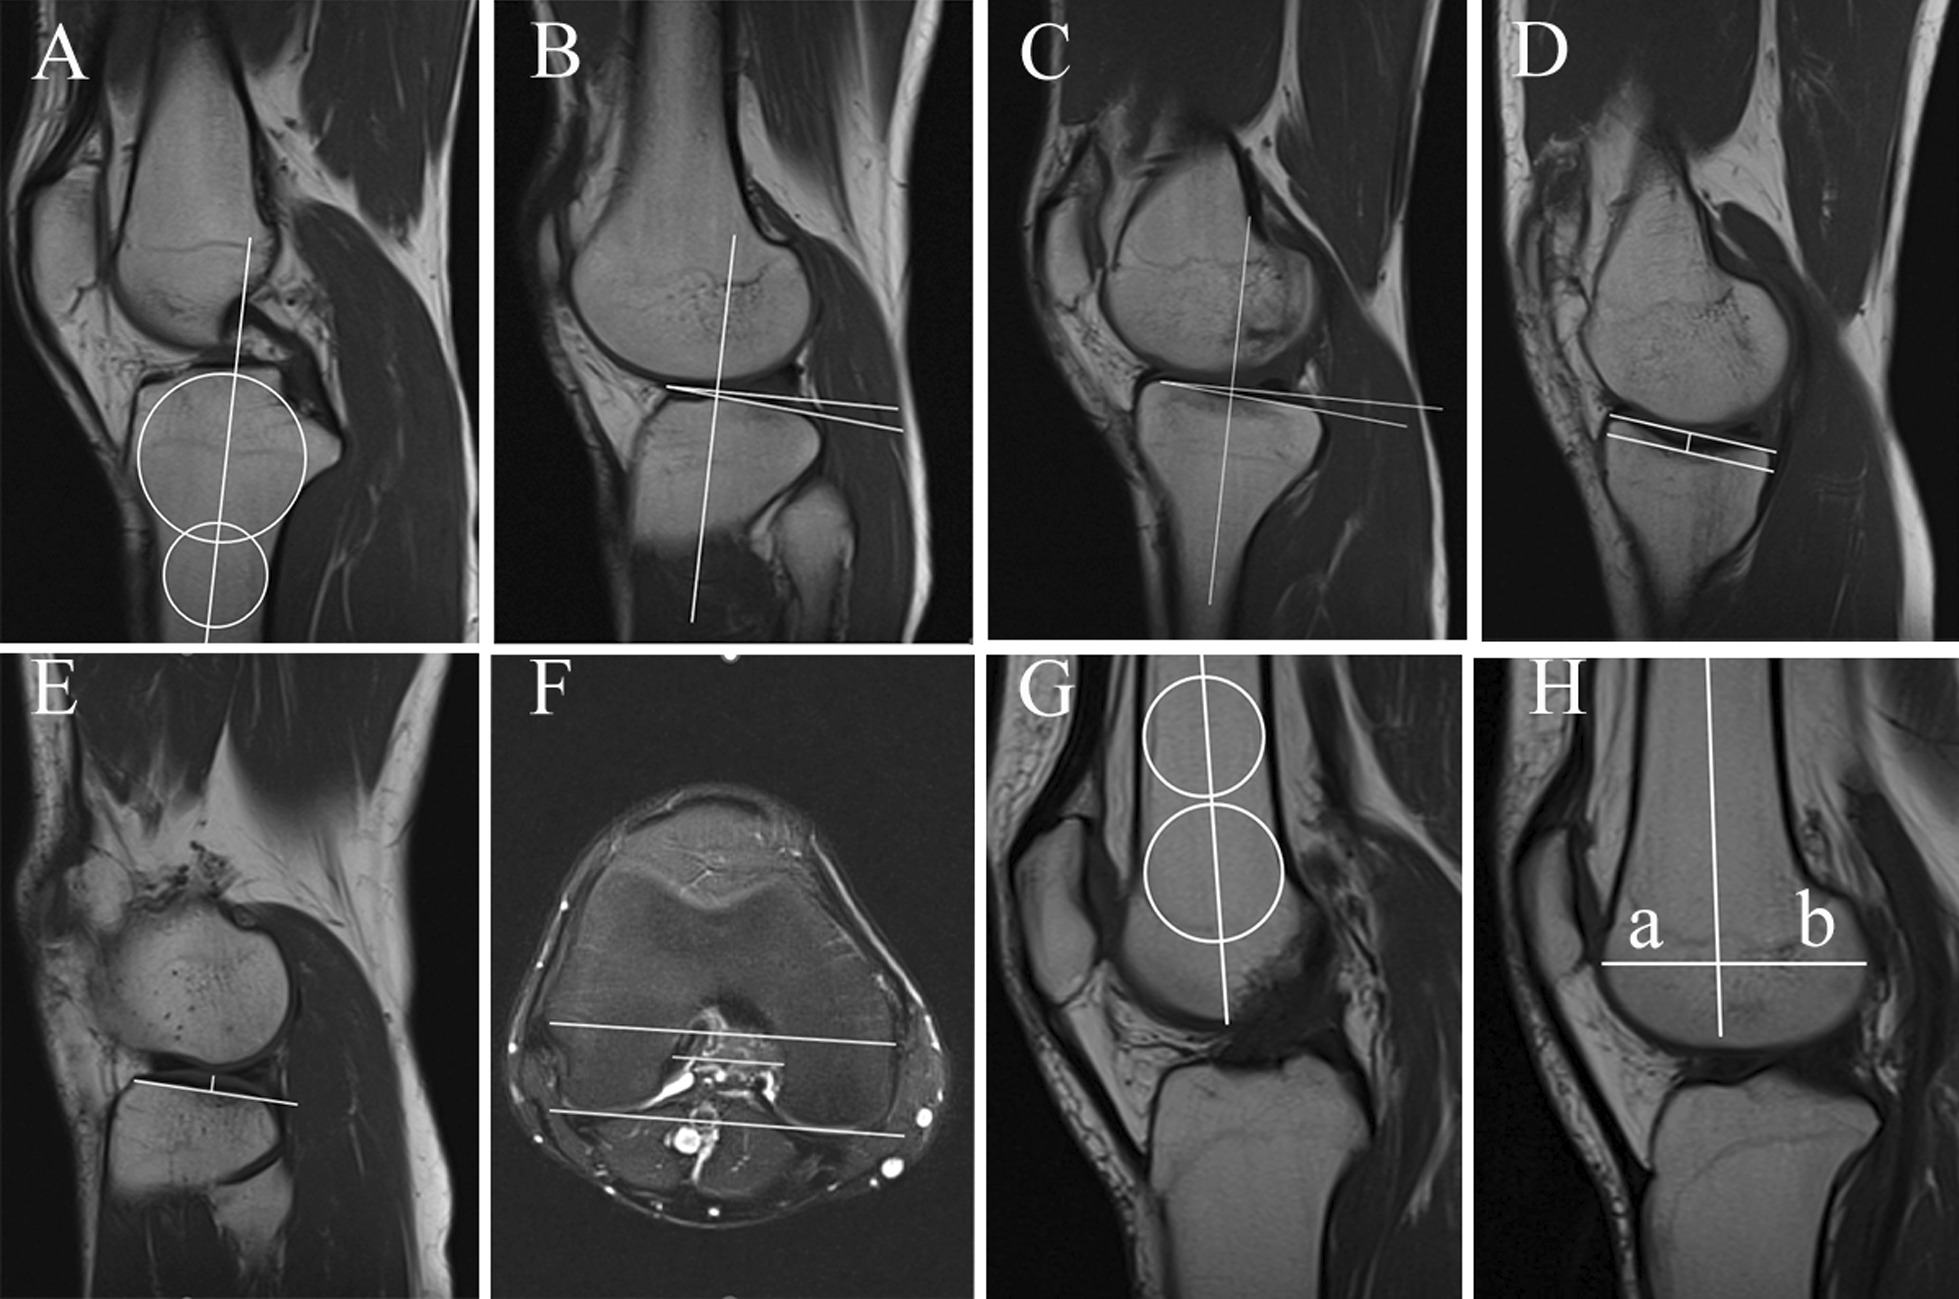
MRI images measurements performed according to the original description. A The anatomic axis of the tibia is determined as the line connecting the centers of 2 circles. B Lateral posterior tibial slope is composed of the vertical line of the anatomic axis of the tibia and the tangent to the lateral tibial plateau. C Medial posterior tibial slope is composed of the vertical line of the anatomic axis of the tibia and the tangent to the medial tibial plateau. D Medial tibial depth. E Lateral tibial height. F Notch width index. G The long axis of the femoral shaft. H Lateral femoral condyle ratio was defined as *b*/(*a* + *b*) × 100%
